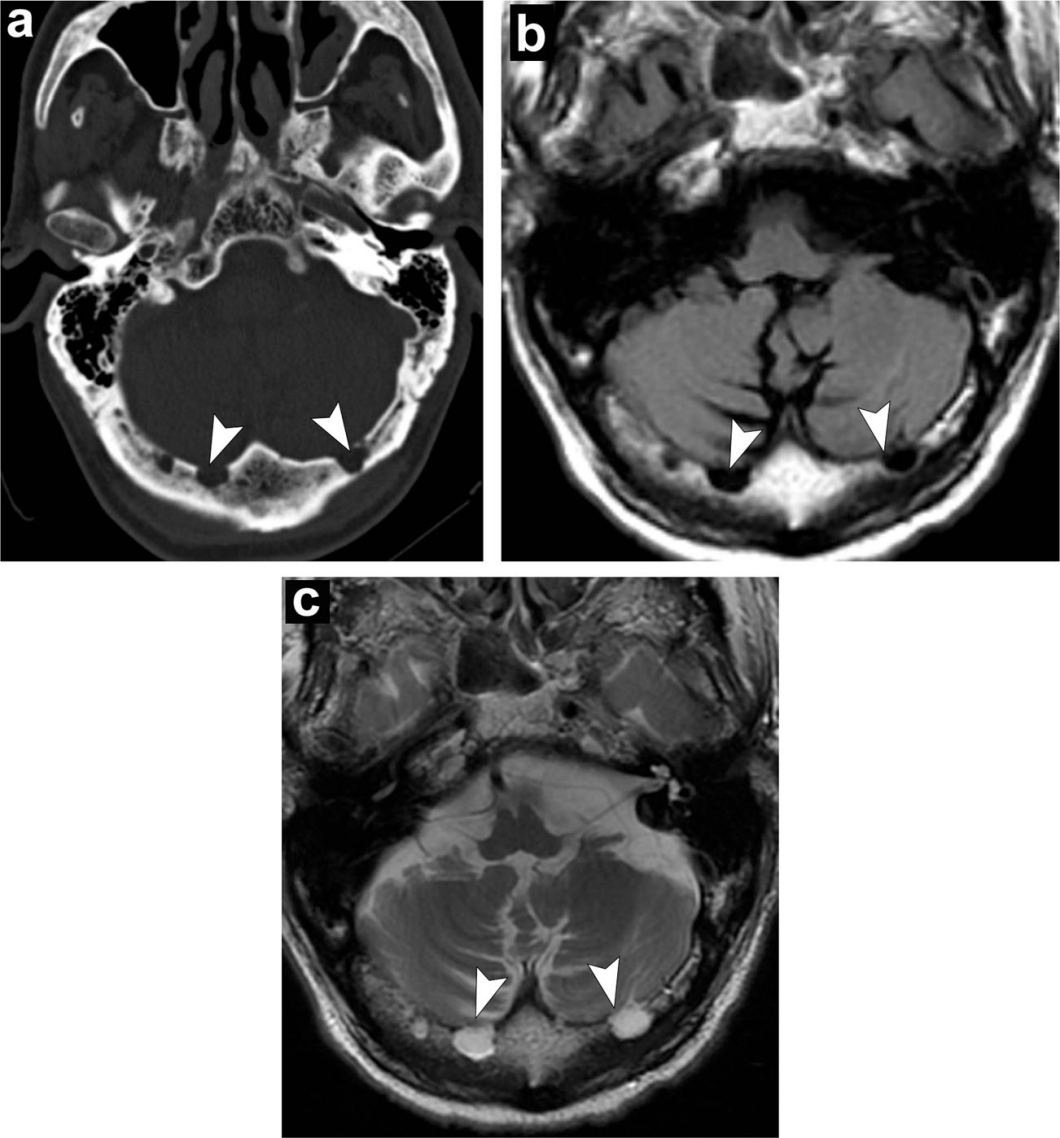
Arachnoid granulations. Axial head CT (a) shows well-defined structures along the inner table in the region of the transverse sinus (*arrowheads*). Axial T1-weighted (b) and axial T2-weighted (c) images demonstrate fluid signal of these structures following CSF along the transverse sinus extending into the occipital bone (*arrowheads*)
